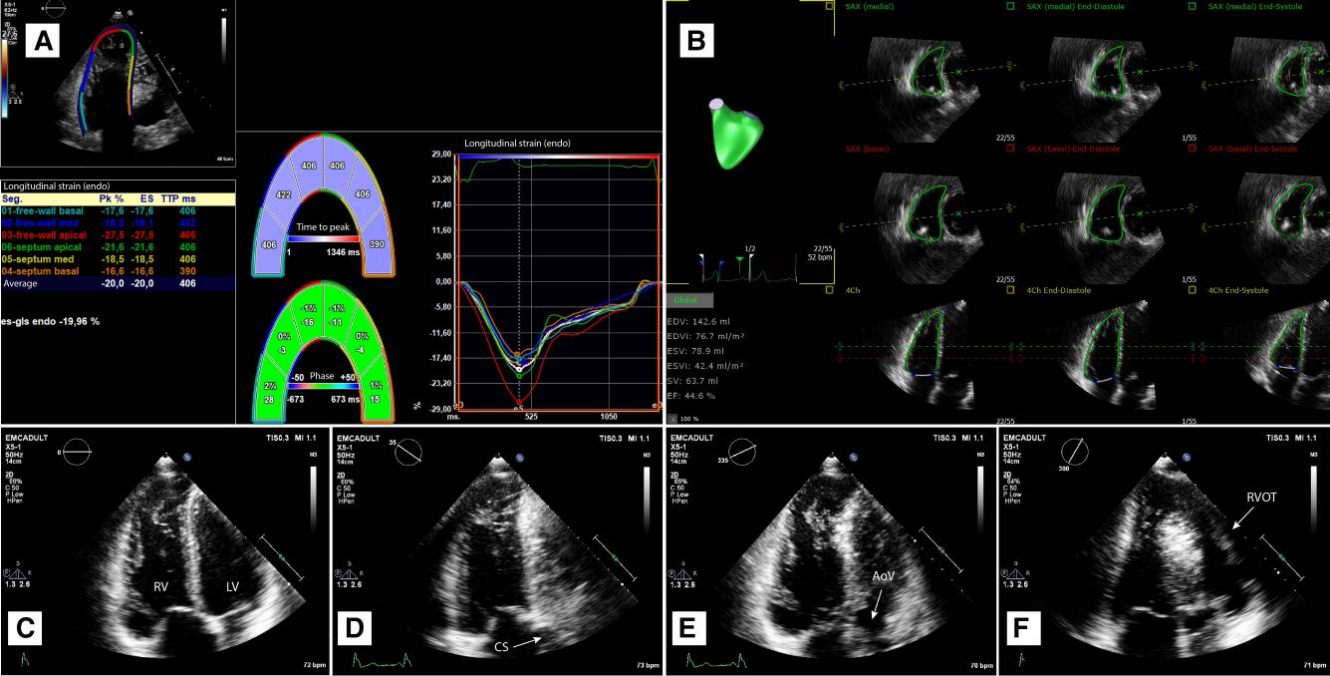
Advanced echocardiographic imaging for the assessment of RV function. Top left panel (*A*)—right ventricular longitudinal strain. Top right panel (*B*)—3D right ventricular ejection fraction. Lower panels (from left to right)—2D multi-plane echocardiography (approximate degrees of electronic rotation, RV wall visualized): C—RV-focused apical four-chamber view (0°, lateral wall); D—coronary sinus view (+40°, anterior wall); E—aortic view (−40°, inferior wall); F—coronal view (−90°, inferior wall and RVOT). Adapted from Bowen *et al*.^9^ with permission. RV, right ventricle; LV, left ventricle; CS, coronary sinus; AoV, aortic valve; RVOT, right ventricular outflow tract.
